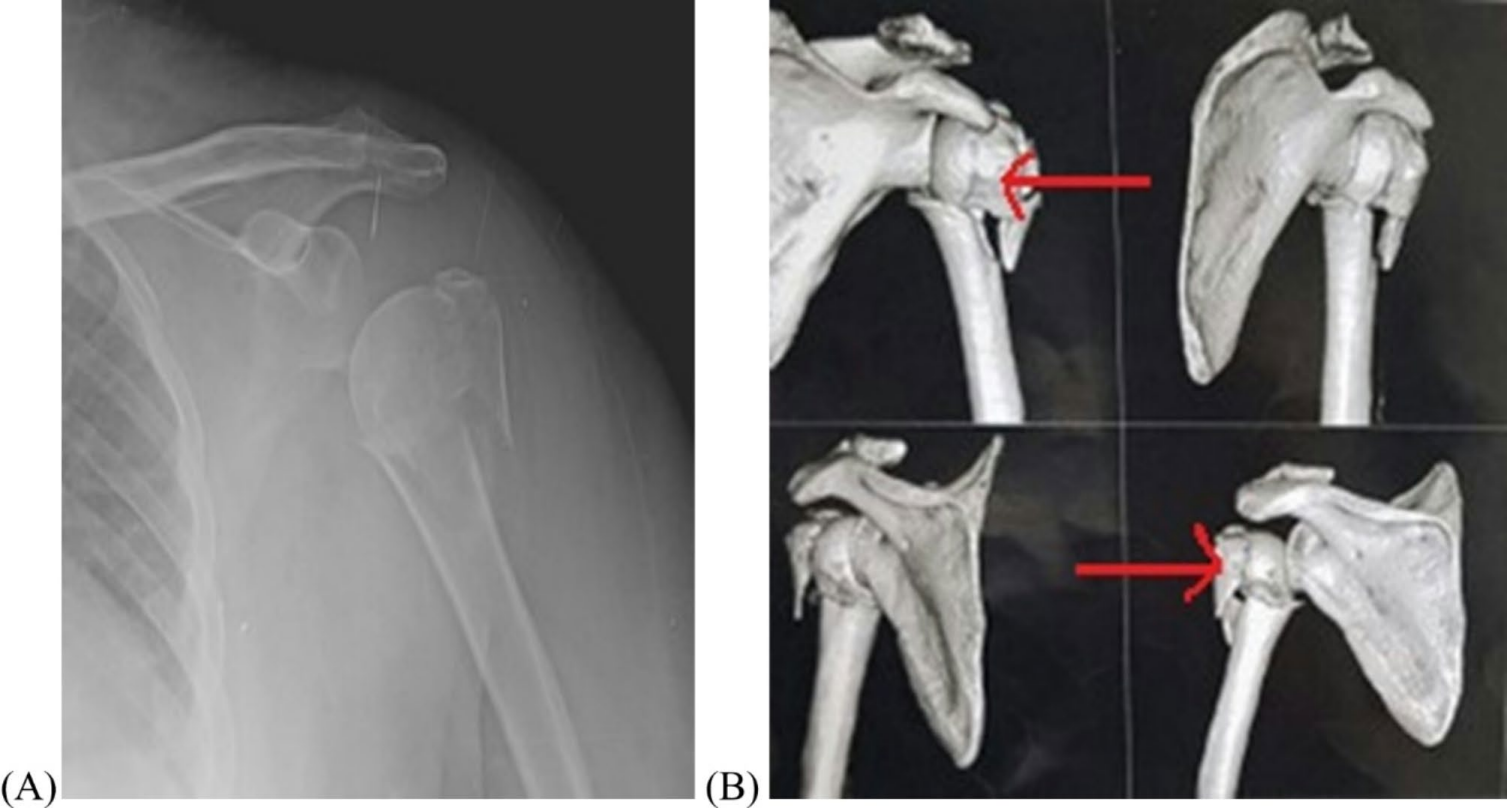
A female patient had a Neer three-part fracture on plain radiograph of the shoulder AP view (A) and CT scan (B) involving greater tuberosity and surgical neck (red arrows)
